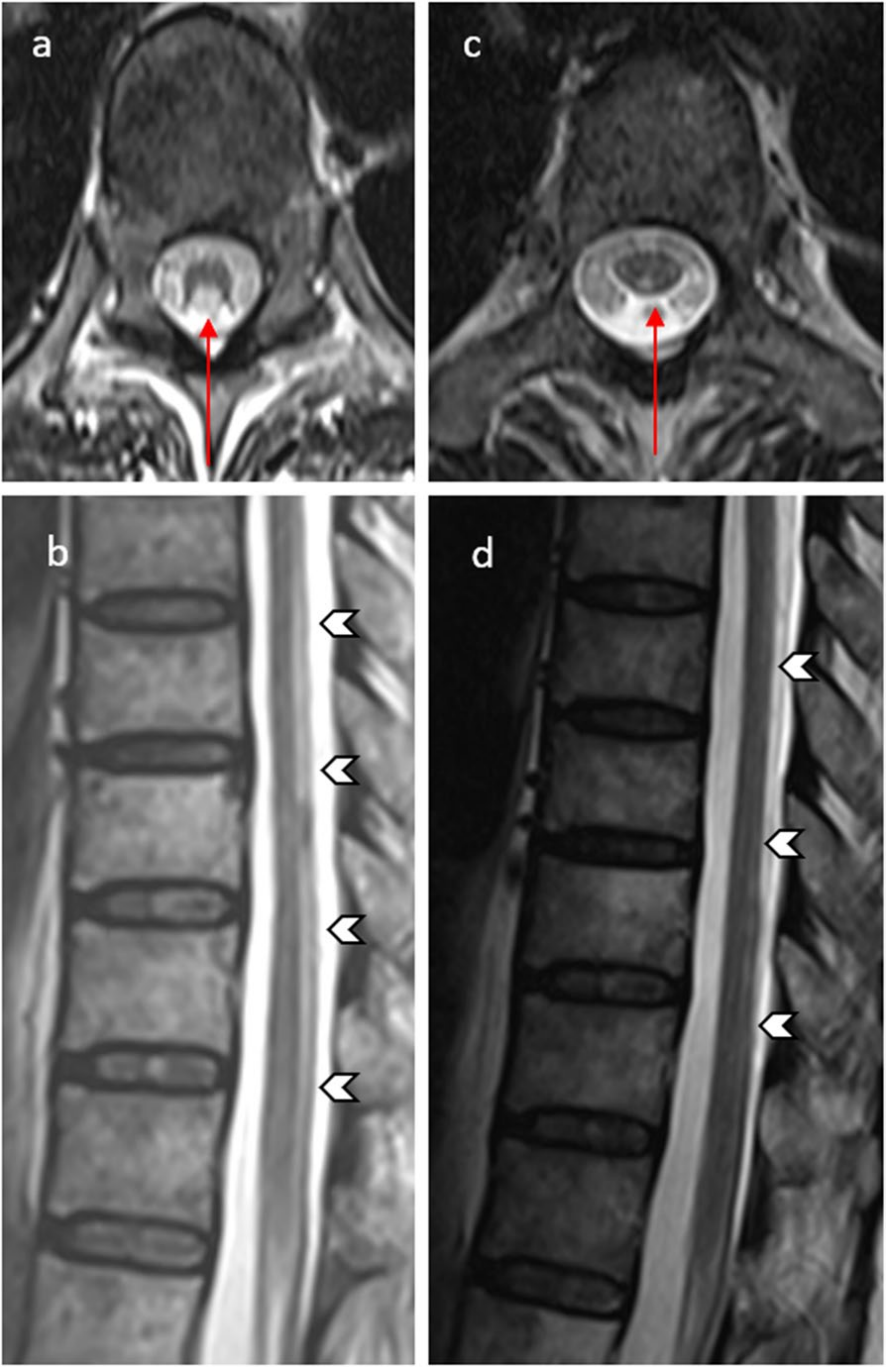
Methotrexate-induced myelopathy mimicking subacute combined degeneration of the spinal cord (SCID). A 54-year-old female with acute lymphocytic leukemia treated with intrathecal methotrexate (MTX) developed bilateral lower limb weakness and numbness approximately 2 weeks after commencing treatment. Axial (a) and sagittal (b) T2-weighted thoracic spine MRI demonstrates high signal lesions involving long segment along the dorsal column of the spinal cord (arrowheads, b). It resembles an “inverted V sign” or “kangaroo ears sign” on the axial image (red arrow, a) masquerading subacute combined degeneration of the cord. Axial (c) and sagittal (d) T2-weighted images at six-month follow-up MRI after cessation of intrathecal MTX treatment show improvement with significant reduction of the signal abnormality within the dorsal column of the spinal cord (red arrow in c and arrowheads in d)
